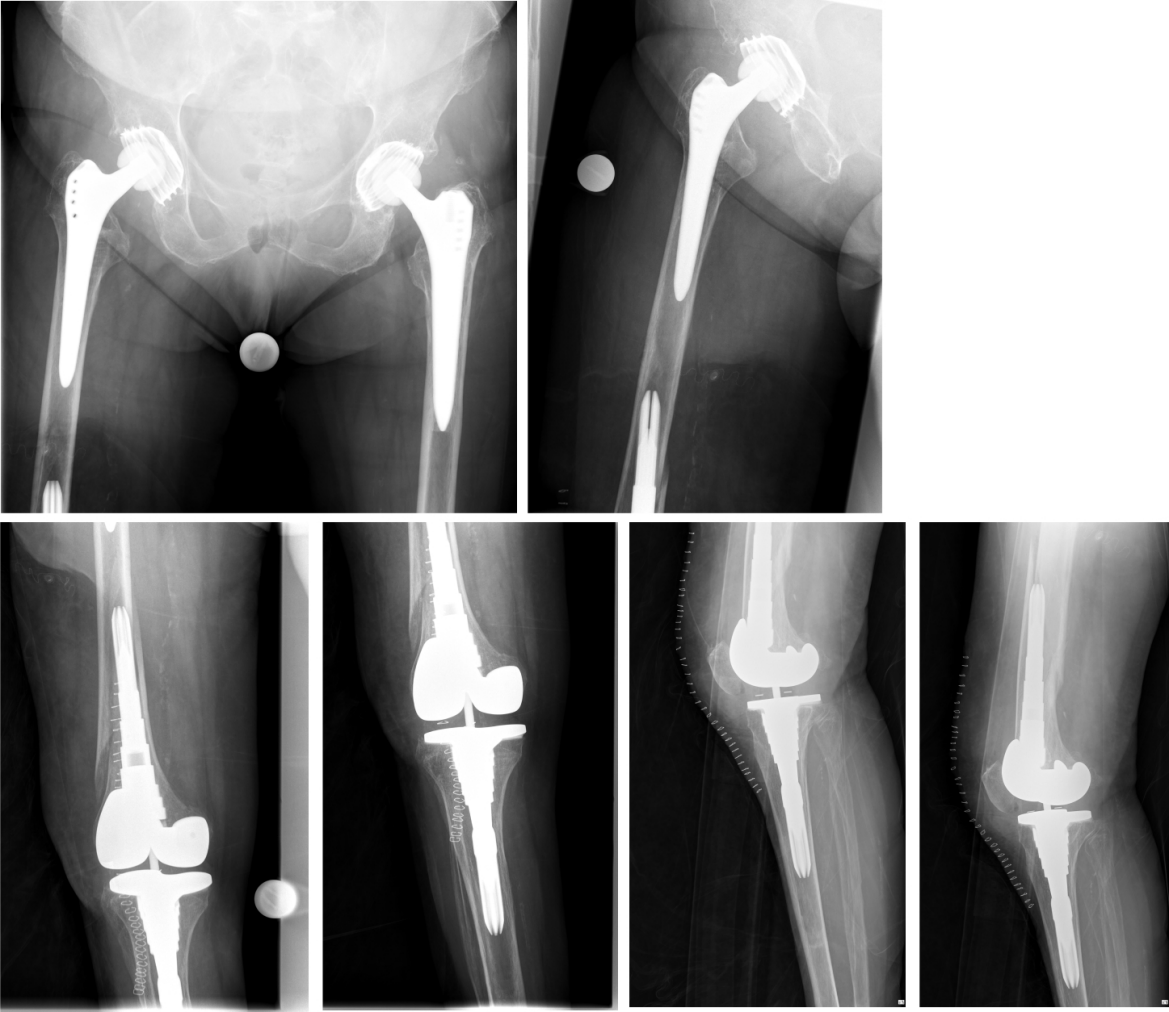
X-ray of the right thigh with the adjacent joints performed immediately after trauma showing the interprosthetic fracture of the femoral shaft
